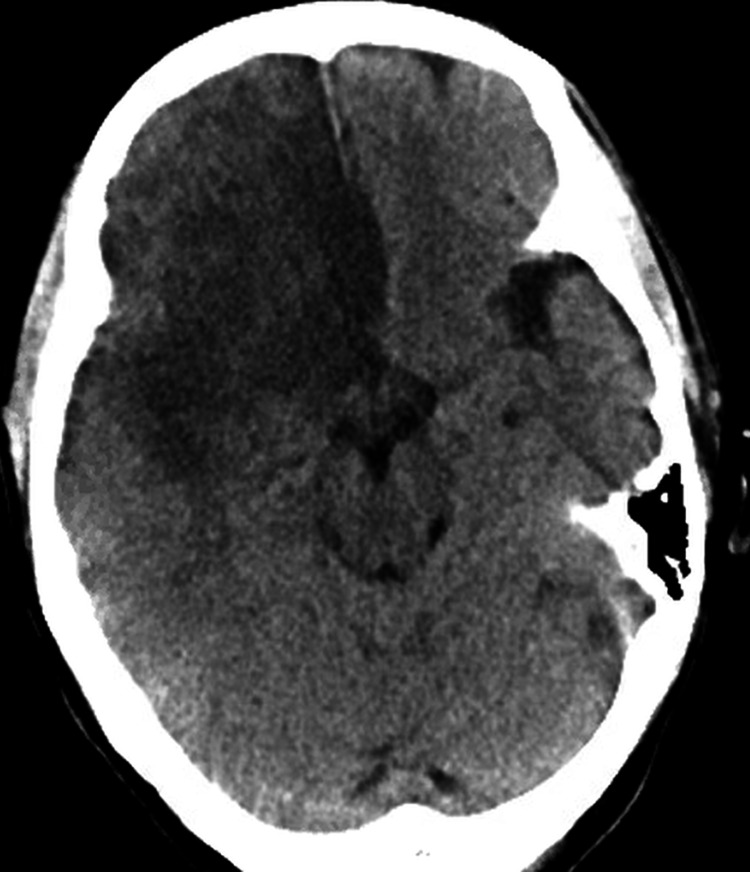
CT head showing right ACA and MCA stroke ACA: Anterior Cerebral Artery, MCA: Middle Cerebral Artery
